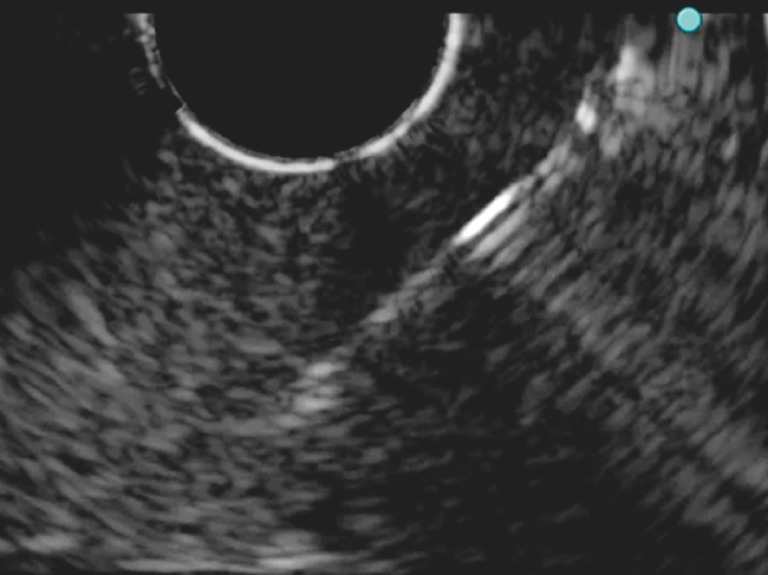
Ultrasound image showing that visibility of the puncture needle improved after correcting the position of the high frequency transducer.
